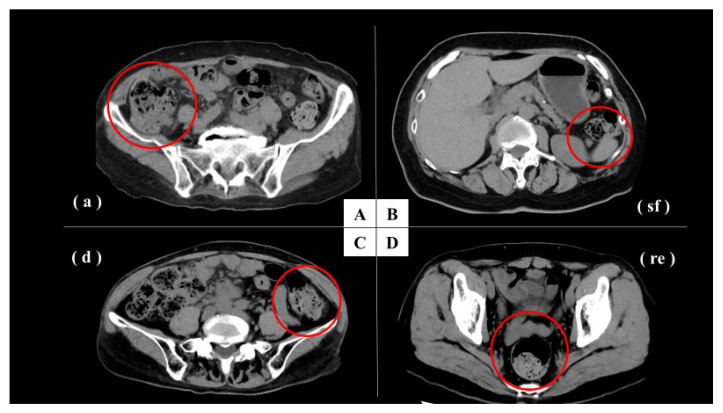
Representative cases. A: CT image was used to rate the stool volume as 4 and the gas volume as 2 (red circle). (a), Ascending colon. B: CT image was used to rate the stool volume as 1 and the gas volume as 2 (red circle). (sf), Splenic flexure. C: CT image was used to rate the stool volume as 3 and the gas volume as 2 (red circle). (d), Descending colon. D: CT image was used to rate the stool volume as 3 and the gas volume as 3 (red circle). (re), Rectum.
